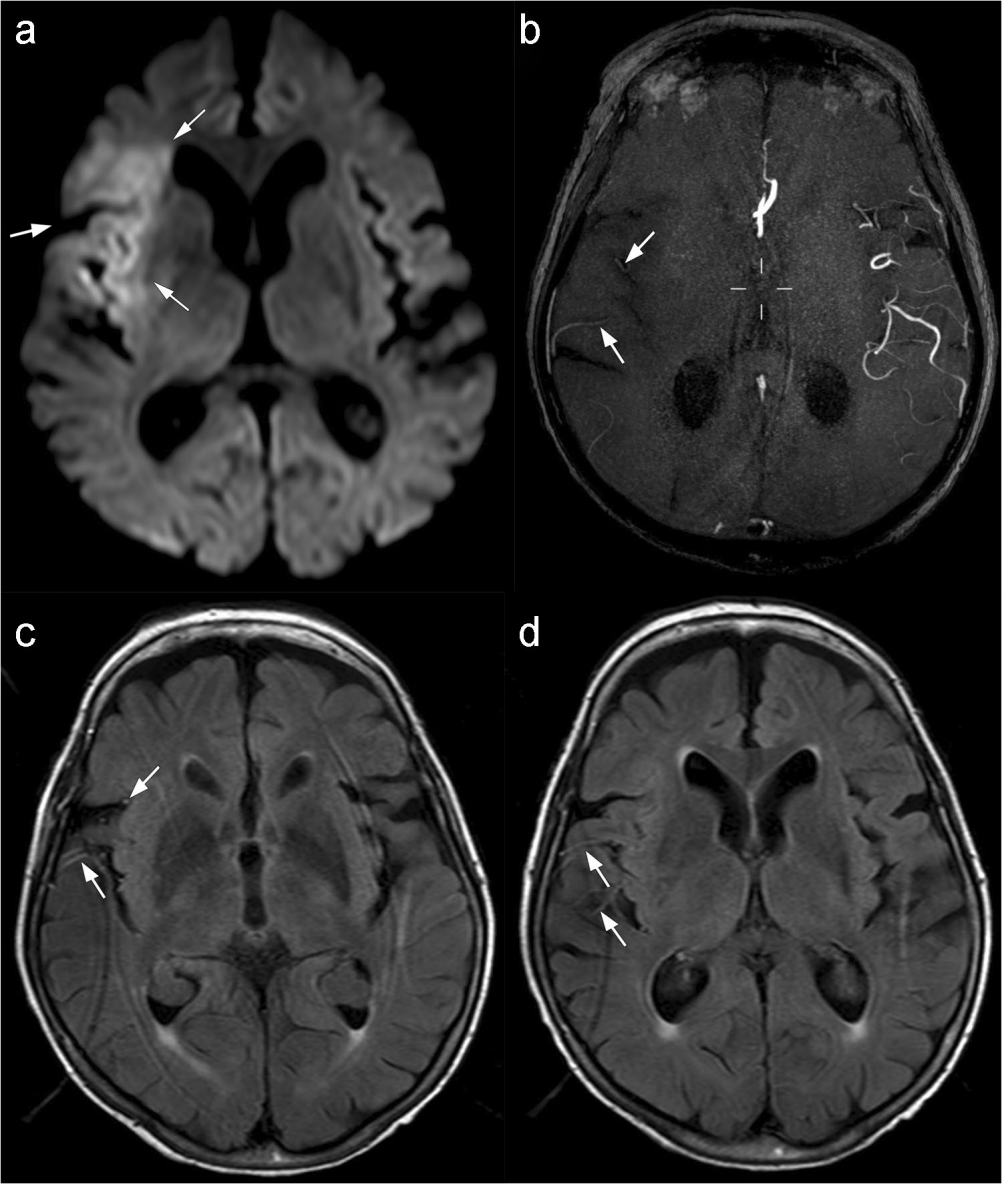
FLAIR hyperintense vessels as a means to assess collateral status. Arrows indicate a abnormal restricted diffusion within an acute ischemic lesion; b collateral vessels visible on time-of-flight MRA, note the paucity of normal middle cerebral artery branches compared with the other side of brain; c and d hyperintense vessels on FLAIR corresponding with MRA collaterals, note also the mismatch between the visibility of the ischaemic brain lesion here compared with image a—this diffusion-FLAIR mismatch is thought to be an indicator of ischemic tissue viability
